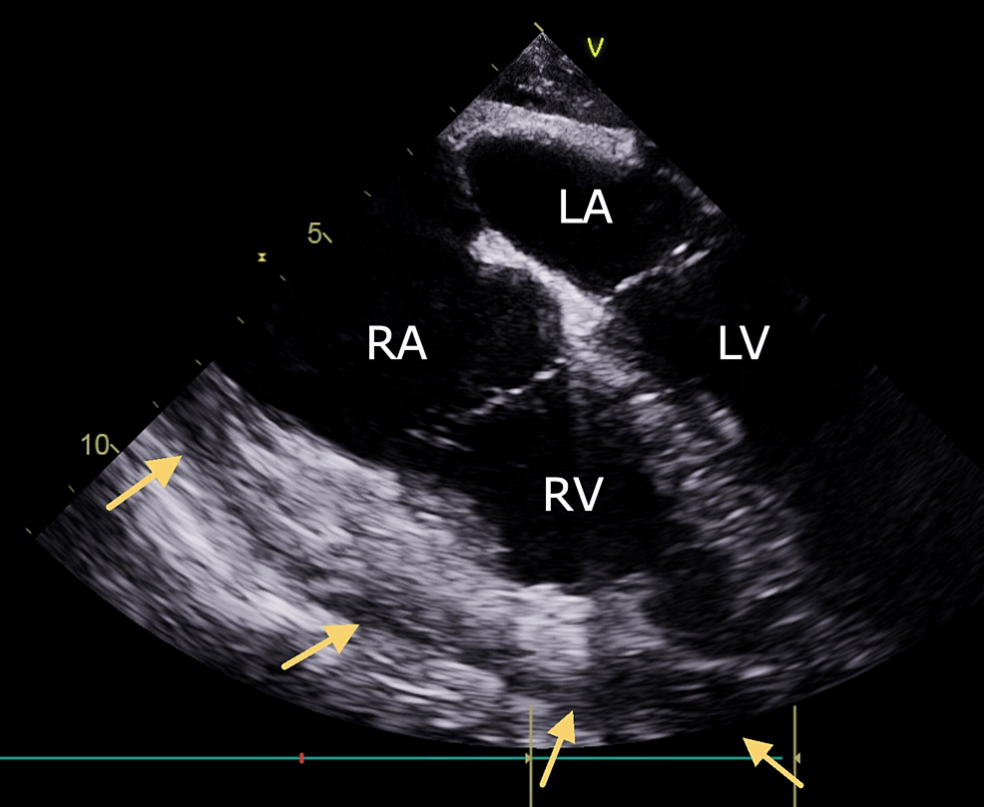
Intraoperative transesophageal cardiac echocardiogram four chamber heart view. Note small pericardial effusion surrounding the myocardium (arrows). RA: right atrium; LA: left atrium; RV: right ventricle; LV: left ventricle.
